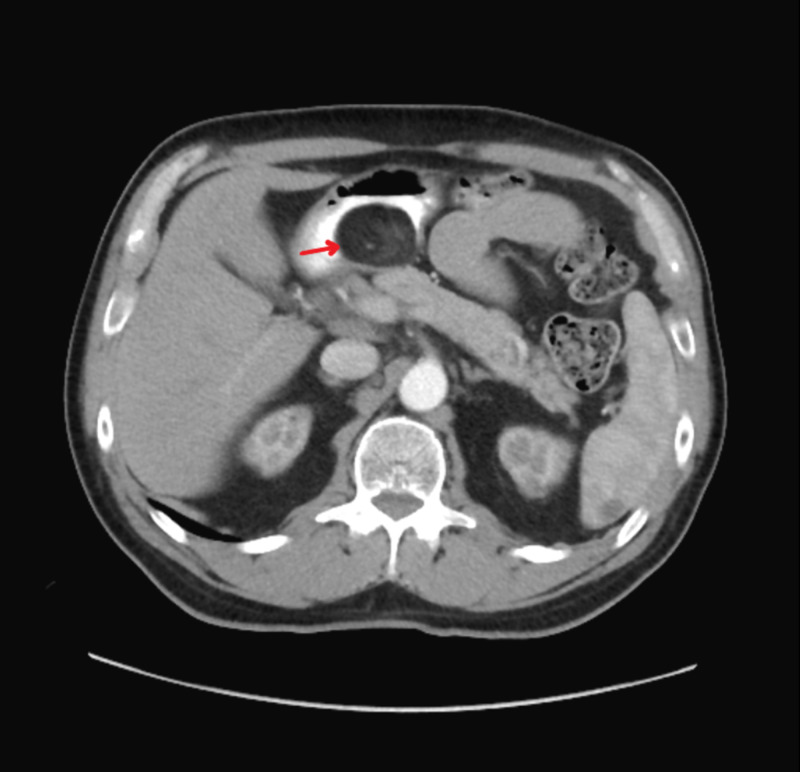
CT image showing a well-circumscribed mass in the lumen of the gastric antrum (arrow - axial view)
